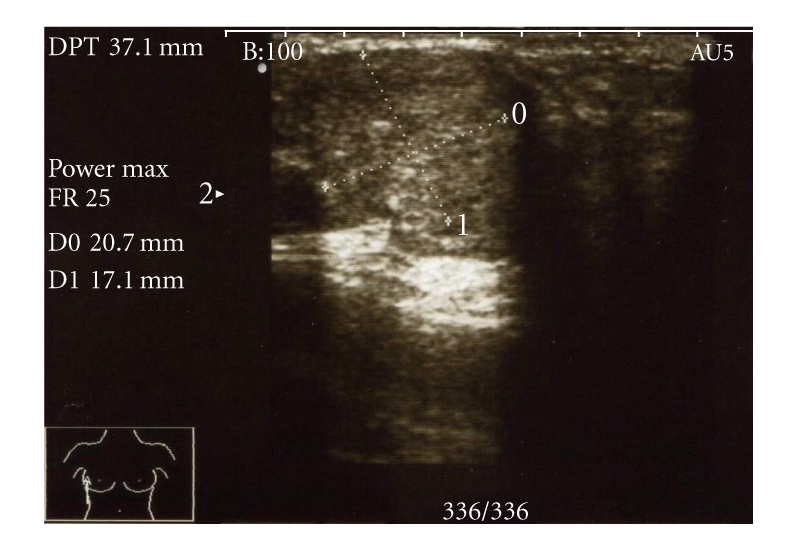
Ultrasound scan of the upper outer quadrant lesion of the right breast.
